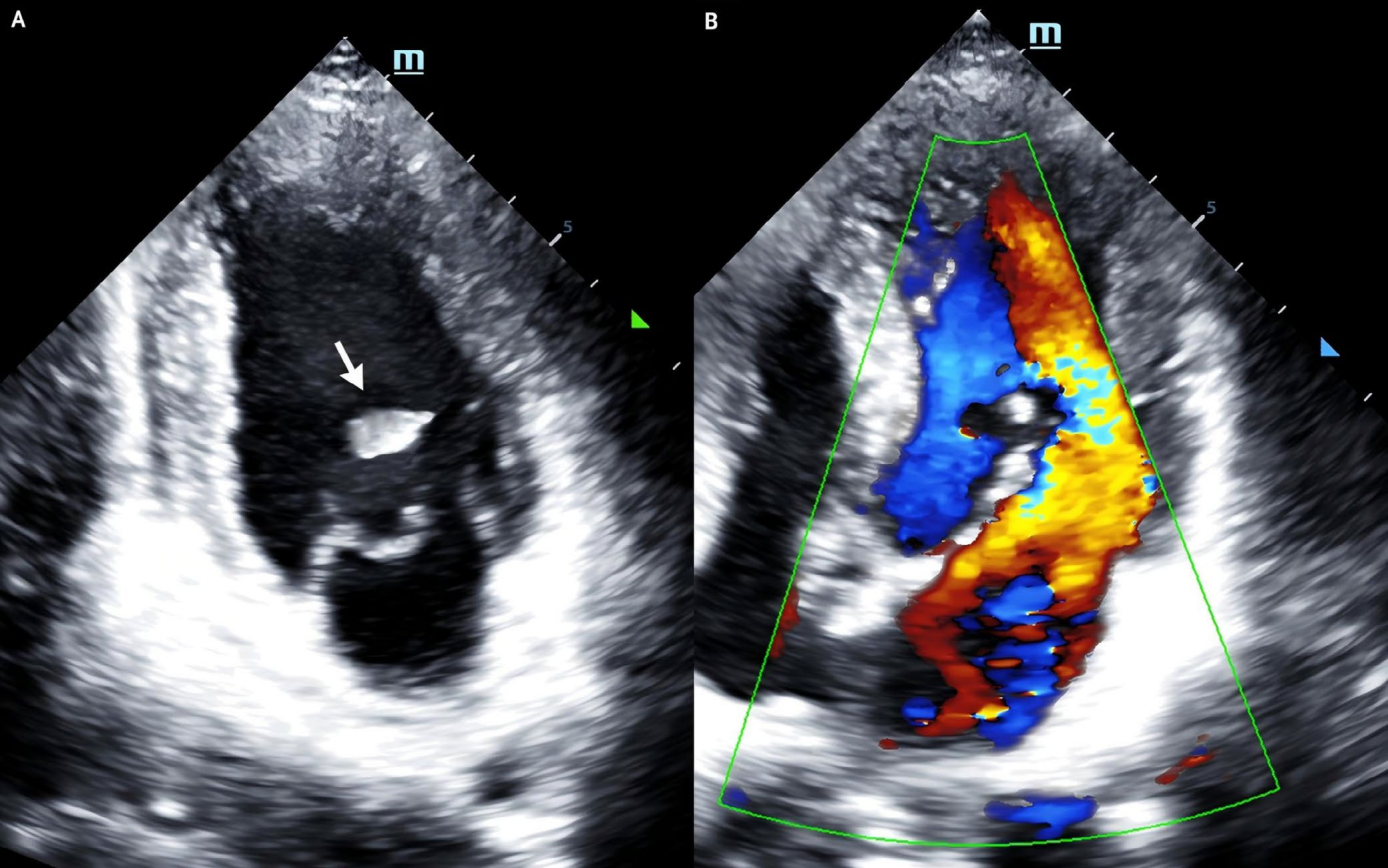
Transthoracic echocardiography showed mitral anterolateral papillary muscle rupture with partial valve body prolapse (A), severe mitral regurgitation (B).
